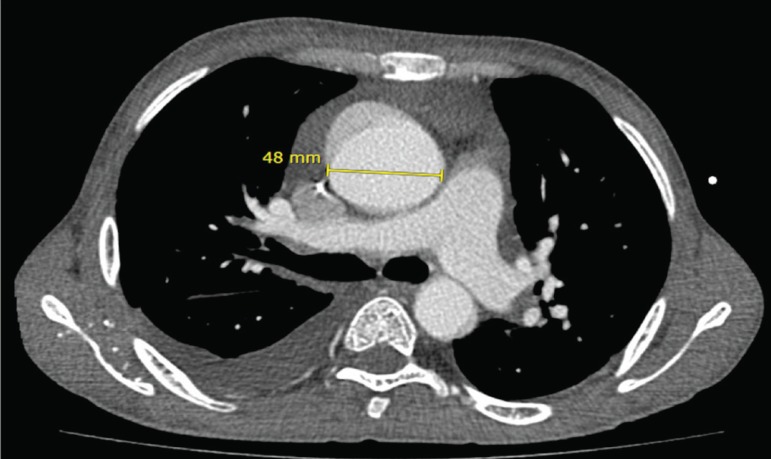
Contrast-enhanced computed tomography transaxial image demonstrating
